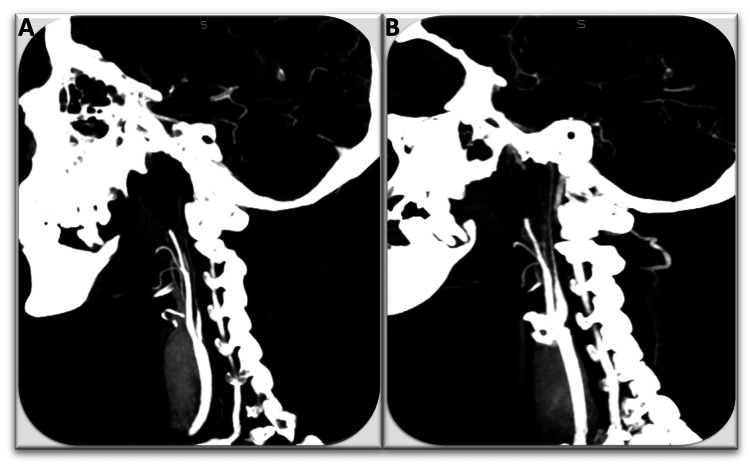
CT angiography of the CAS: filiform appearance of the internal carotid arteries. (A) right carotid and (B) left carotid CT: computed tomography, CAS: carotid artery stenosis
